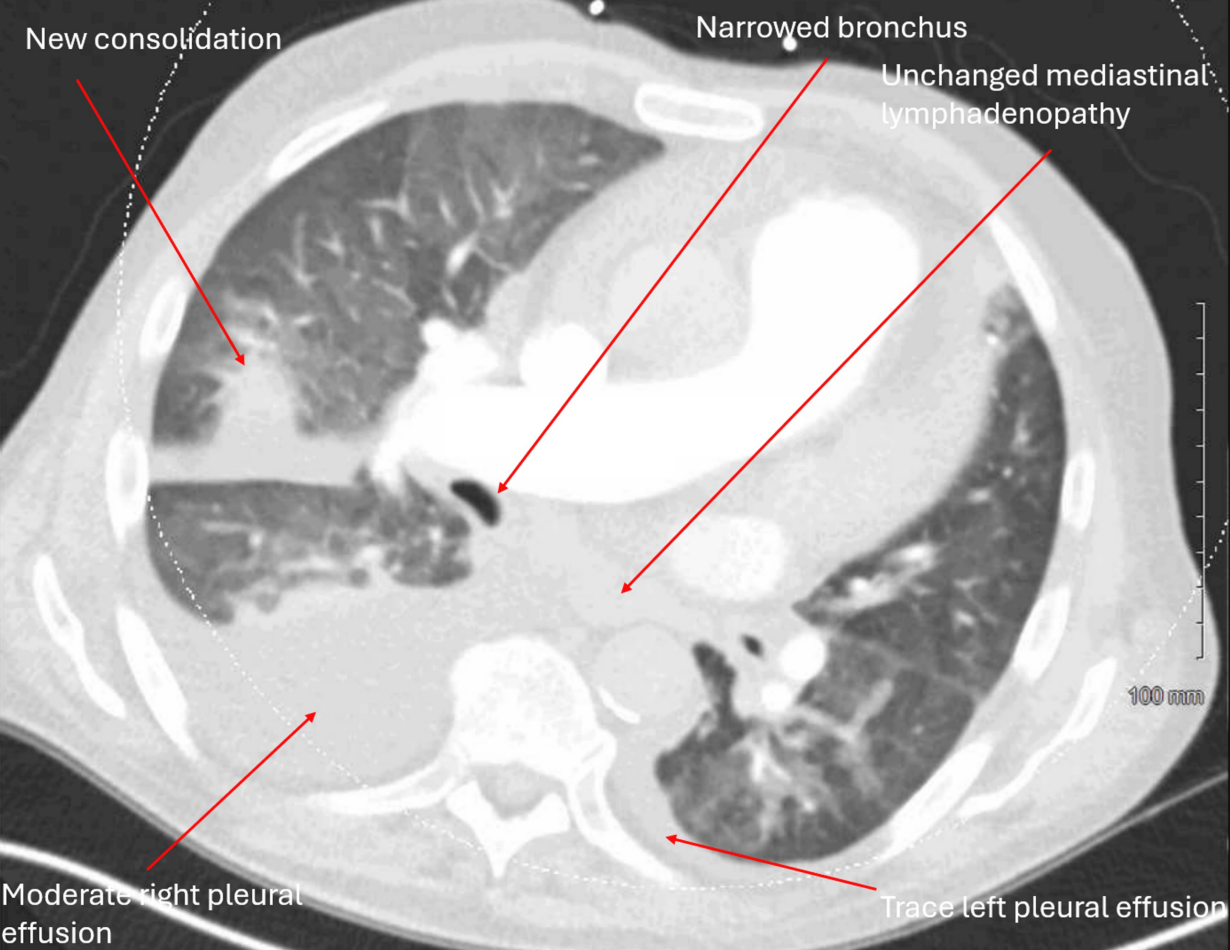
CT pulmonary angiogram with IV contrast on 10/24/22 showing no evidence of PE, a new consolidation in the right lower lobe, improving right middle lobe mass-like consolidation with persistent narrowing of the right middle lobe bronchus, increasing moderate right pleural effusion, small left pleural effusion, and unchanged mediastinal lymphadenopathy.
